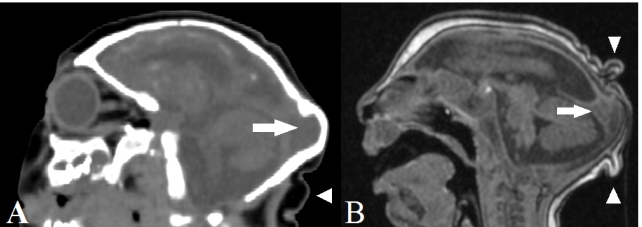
Sagittal computed tomography (A) and T1-weighted
